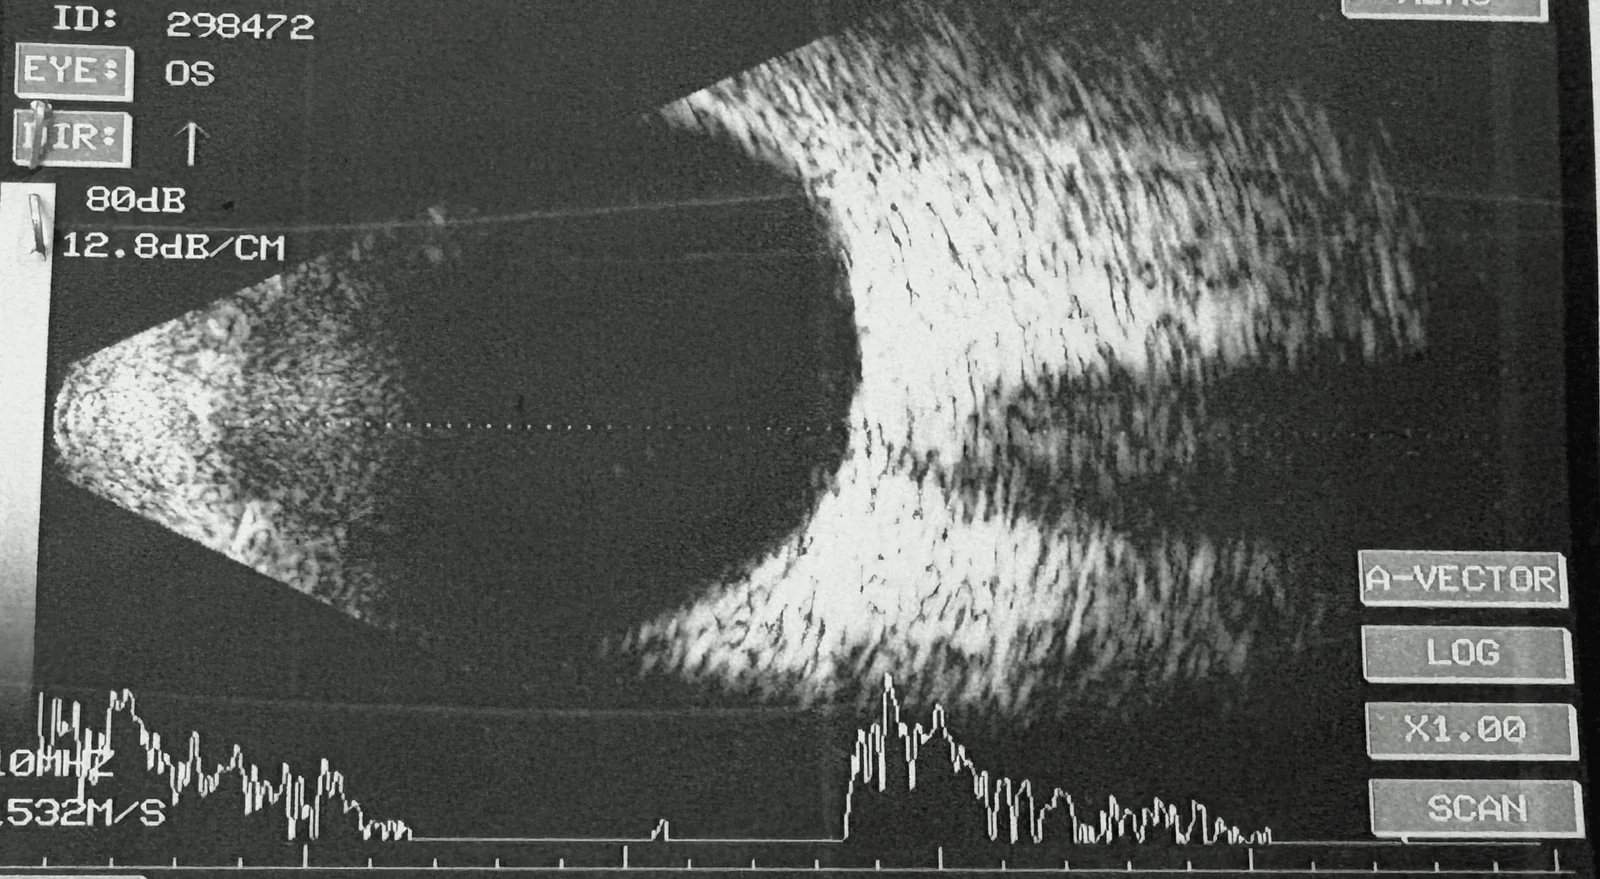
B-scan ultrasonography of the left eye showing a normal posterior segment.
